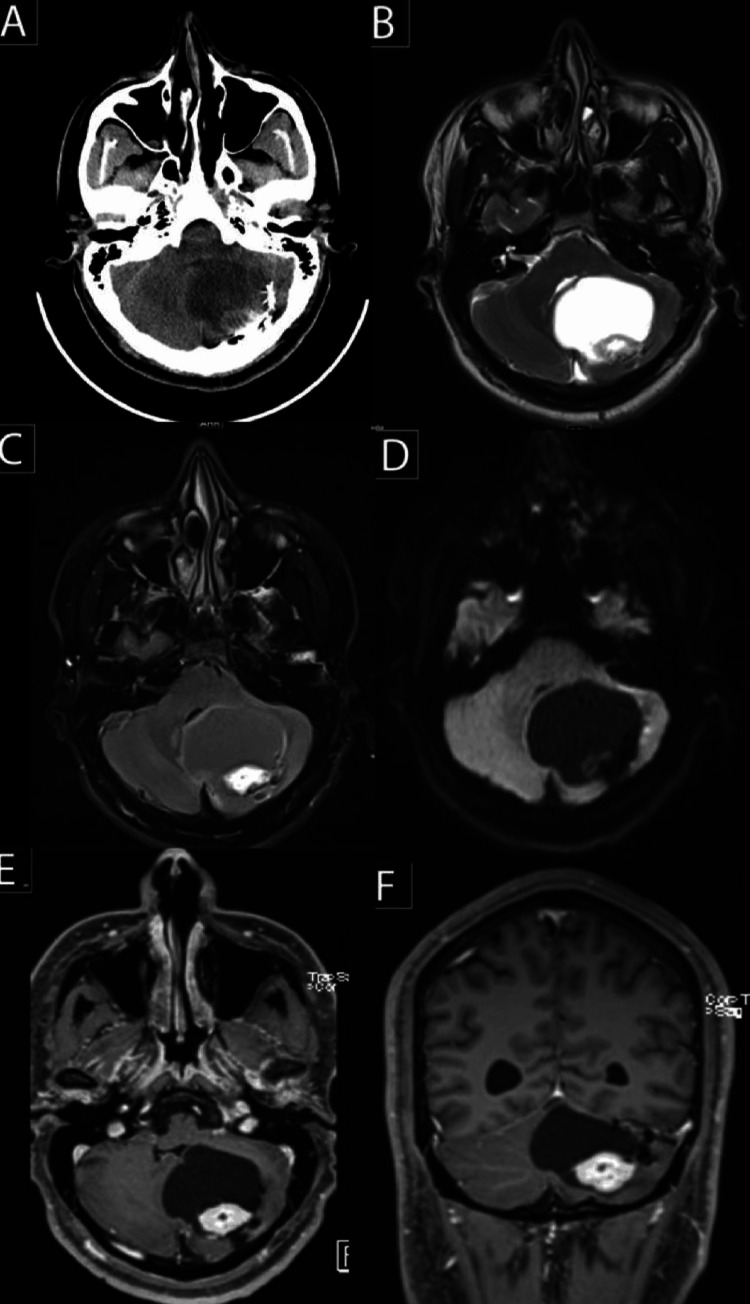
(A) CT brain without contrast. (B) T2-weighted image. (C) FLAIR. (D) Diffusion-weighted image. (E) Axial T1 post-contrast. (F) Coronal T1 post-contrast. FLAIR: Fluid-attenuated inversion recovery.
